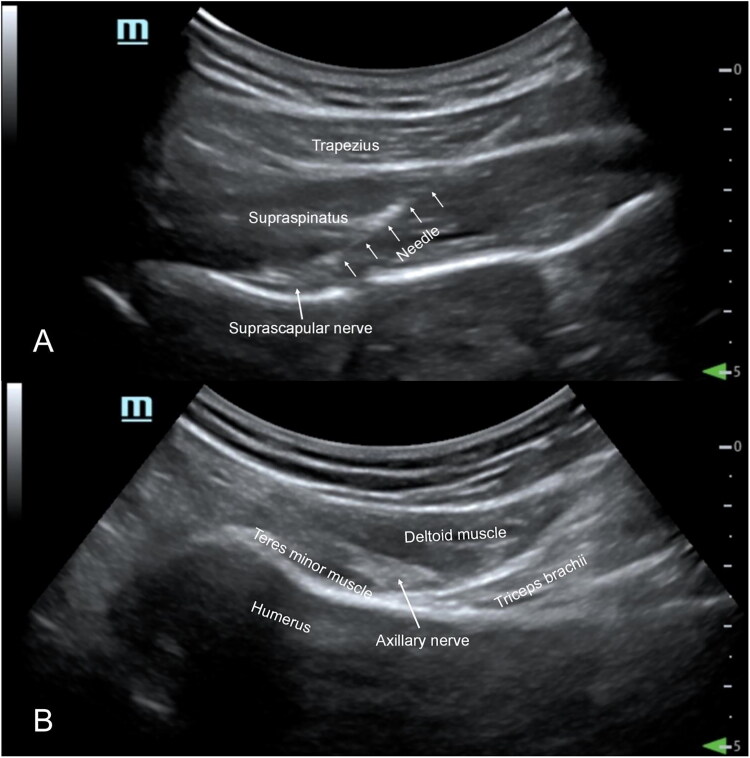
Ultrasound-guided puncture of SSN (A) and an (B). Note: SSN, suprascapular nerve; AN, axillary nerve.
